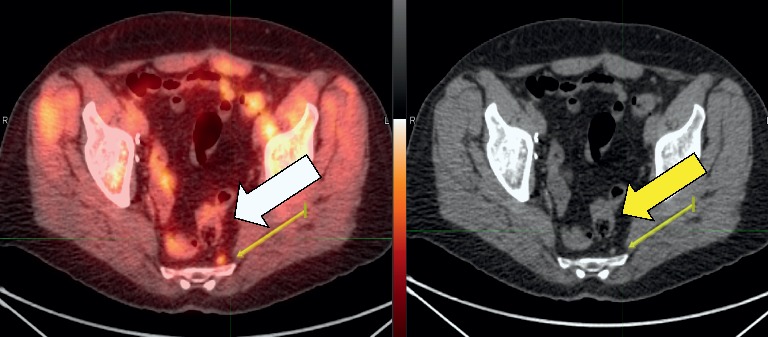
Images of a 68-year-old male with T3N0M0 prostate cancer. He had undergone radical prostatectomy, bilateral lymph node dissection, and salvage radiation therapy. He was referred for ^18^F-fluciclovine imaging with PSA rising to 0.7 ng/mL. The axial, fused PET/CT image on the left identifies a focus of enhanced ^18^F-fluciclovine uptake (white arrow) in the presacral region. The corresponding CT image on the right identifies a 6-mm lymph node metastasis (yellow arrow).
